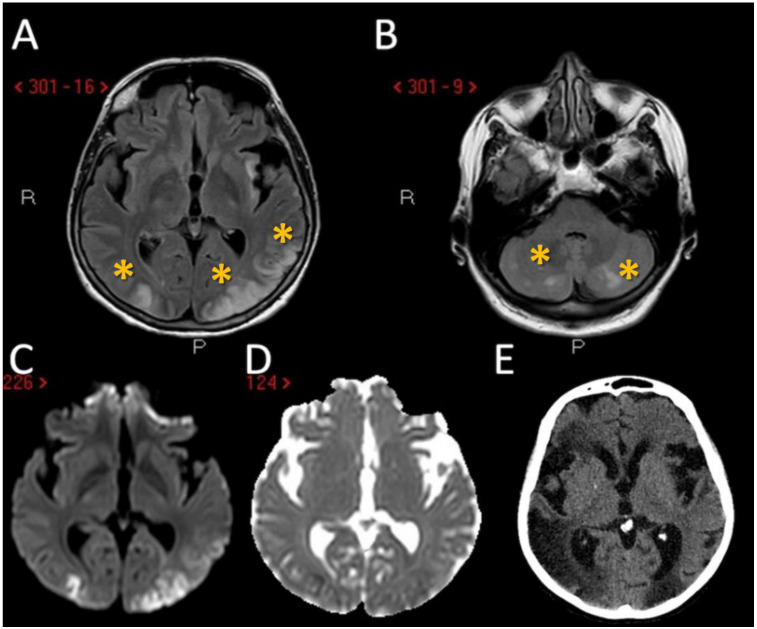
Neuroimaging studies of the third patient. MRI of patient 3 showed the presence of multiple supratentorial (A) and infratentorial (B) hyperintense lesions (yellow asterisk) with a territorial distribution in A and border zone pattern in B (axial FLAIR sequences) with DWI and ADC pattern suggesting acute ischemic lesions with a metachronous timing pattern (C,D). In the follow-up CT performed 7 days later it is evident the increased number of ischemic lesions, in particular on the right hemisphere (E).
